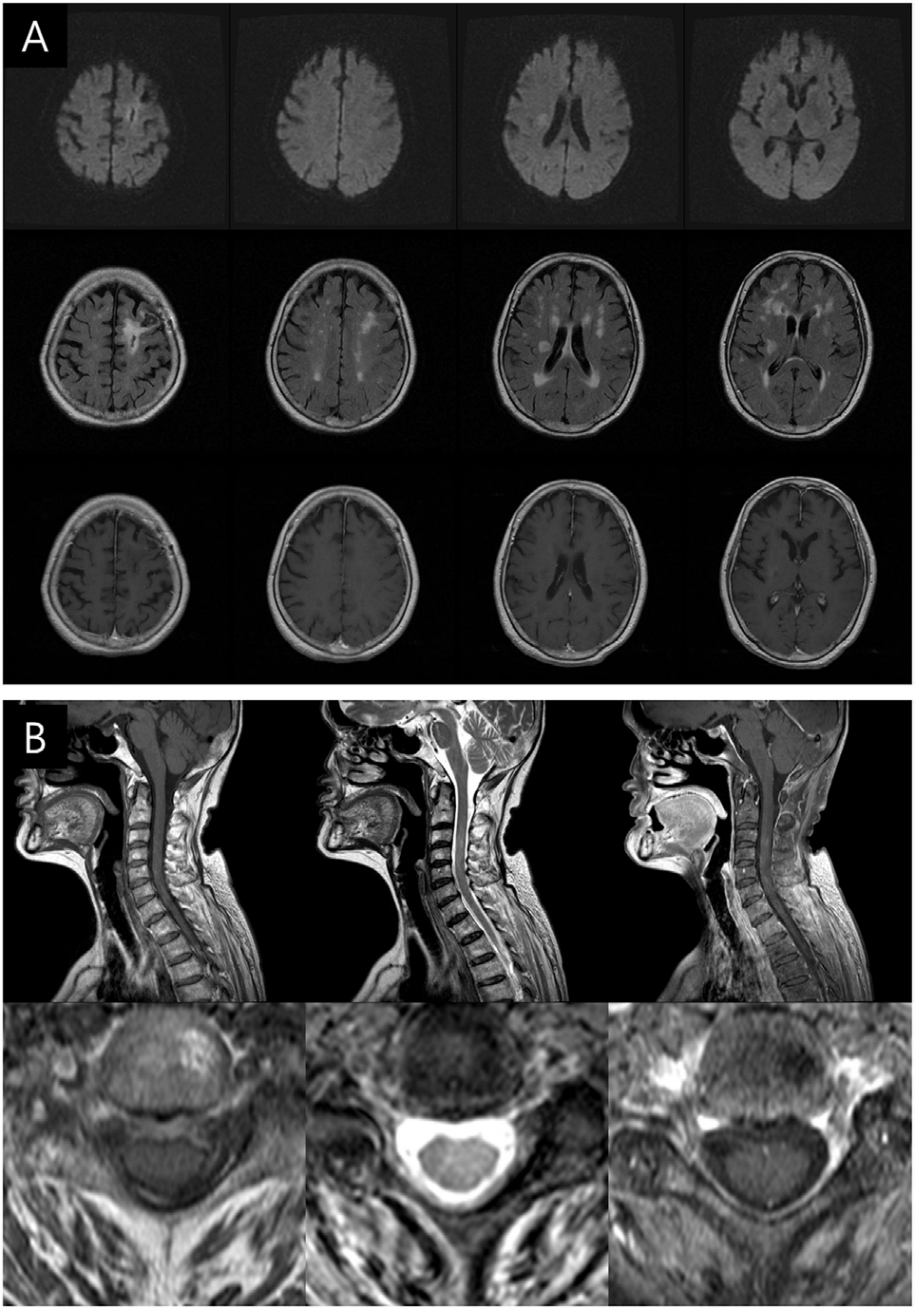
Follow-up brain and cervical spine magnetic resonance imaging (MRI) scans taken 3 months after the maintenance of antibiotic treatment. (A) Diffusion-weighted imaging (upper row), T2 fluid attenuation inversion recovery (middle row), and enhanced T1-weighted axial images (lower row) showing marked decrease in size of multiple lesions and perilesional edema. (B) Cervical spine MRI (T1, T2, and enhanced T1) displaying the nearly disappeared of high-intensity lesions in the cervical spinal cord.
